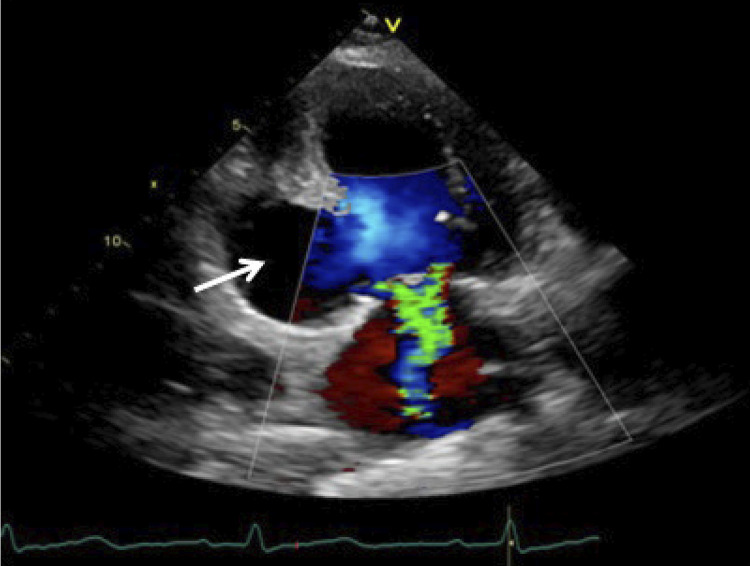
Ecocardiografia transtorácica (incidência apical 2 câmaras) evidenciando formação aneurismática (seta).
